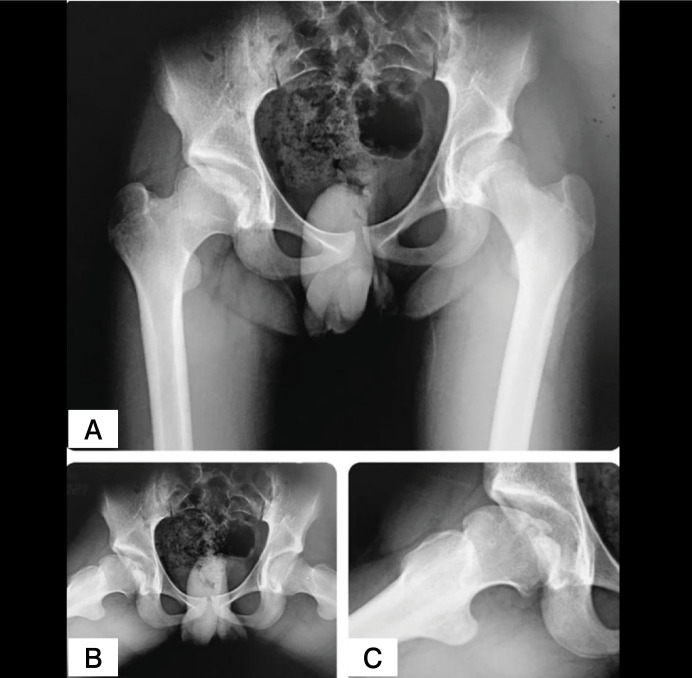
Pelvis AP (A), Frog-leg (B) and close view (C) of the right hip in the Frog-leg view radiographs. Note an osteochondral fragment in the central part of the femoral head. In the Frog-leg view, the fragment appears to be detached from its bony base.
